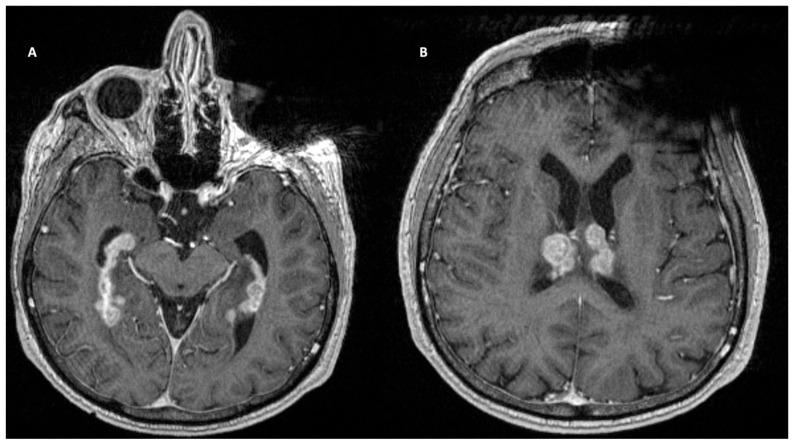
T1-weighted contrast-enhanced MRI demonstrated a large B-cell lymphoma centered in the subependymal regions of the lateral ventricles.
